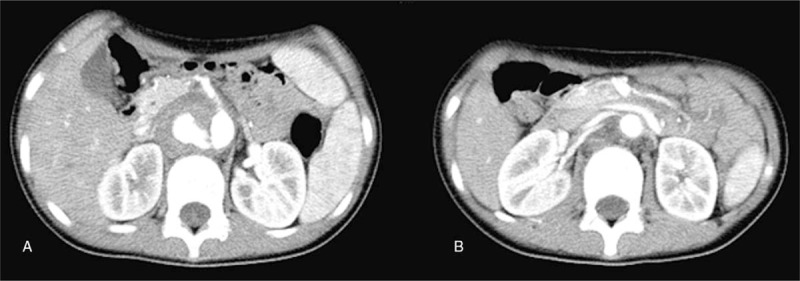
CTA: A crevasse could be seen on the right side of the abdominal aorta at the level of the superior mesenteric artery because of leaking contrast medium (A). Stenosis is seen at the beginning of 2 right-kidney-feeding arteries, which originated from the abdominal aorta and tortuous left-kidney artery (B).
